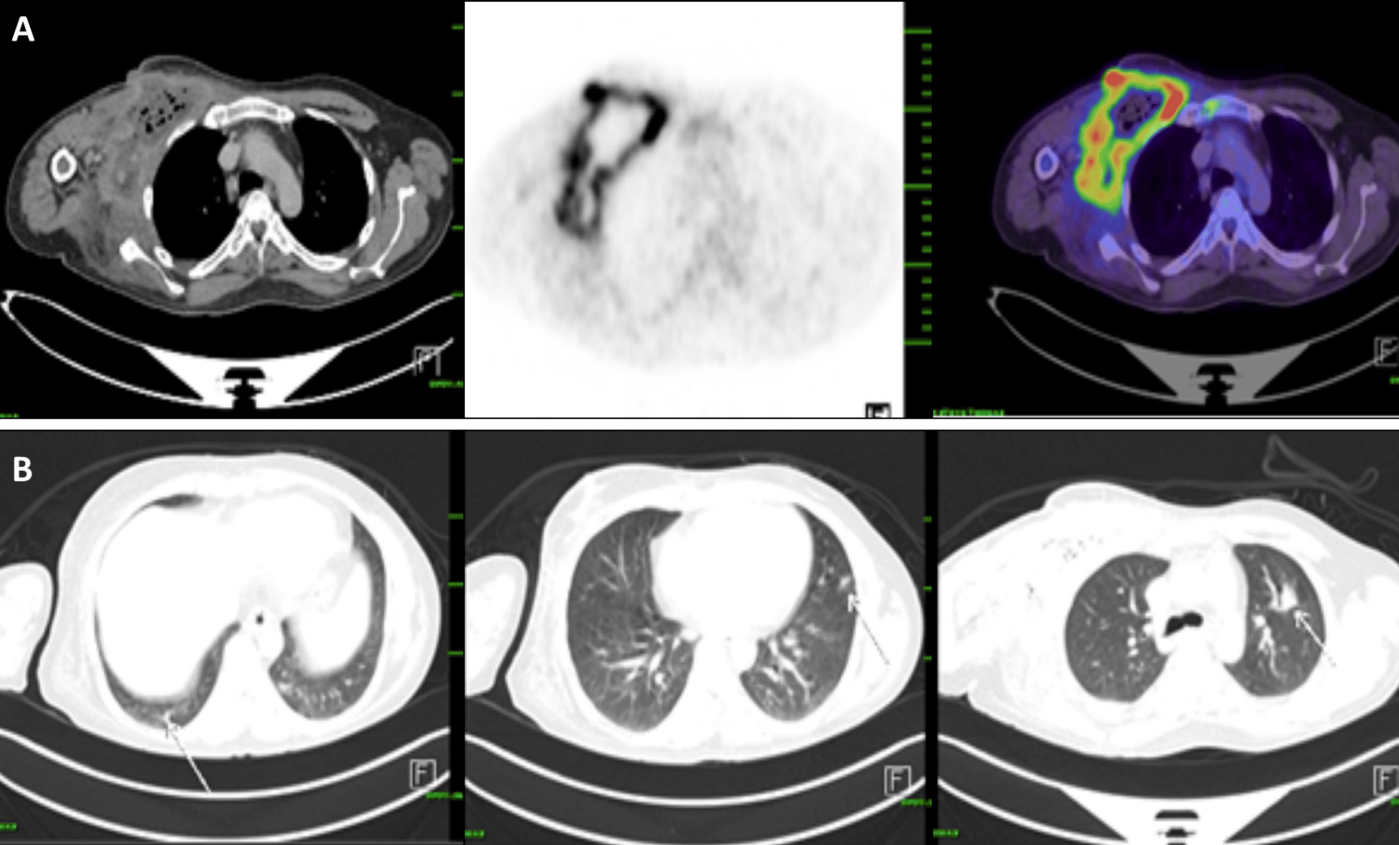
PET-CT Images Post Three Cycles of R-Chop Regimen. (A) A large ill marginated peripherally enhancing, peripherally FDG avid (SUV max 13, previously 20.0) necrotic mass lesion with multiple air foci within measuring ~ 5.6 × 10.5 cm (previously ~ 14 × 17.5) is seen involving the intermuscular plane of right anterolateral chest wall, seen extending up to skin – significant decrease in the size and metabolic activity is noted. (B)Significant reduction in the size with complete resolution of metabolic activity of the multiple FDG-avid nodules in bilateral lungs, measuring ~ 7 × 7 mm (previously 29 × 22 mm) in the posterior segment of the right lower lobe.
